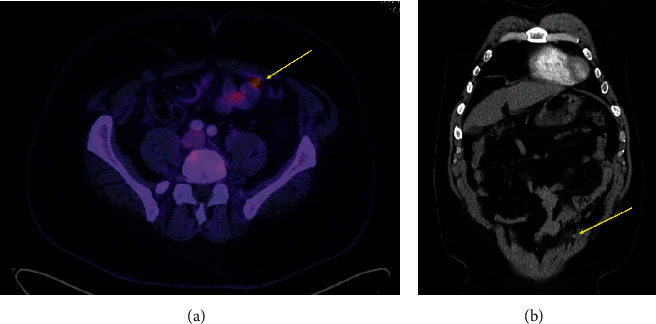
(a) Axial image of PSMA PET showing abdominal wall mass concerning for recurrence. (b) Coronal image of CT scan showing small abdominal wall mass correlating with the mass on PSMA PET scan.
